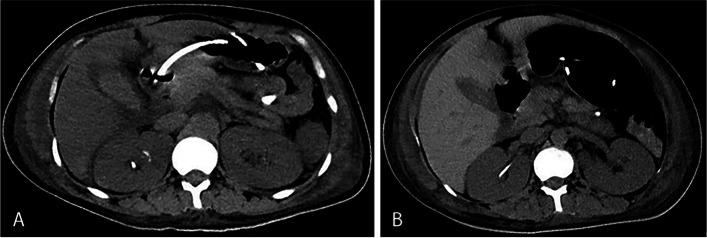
Computed tomography scan of both kidneys. A Bilateral renal and perirenal conditions at the first week after admission. B Bilateral renal and perirenal conditions at the third week after admission
